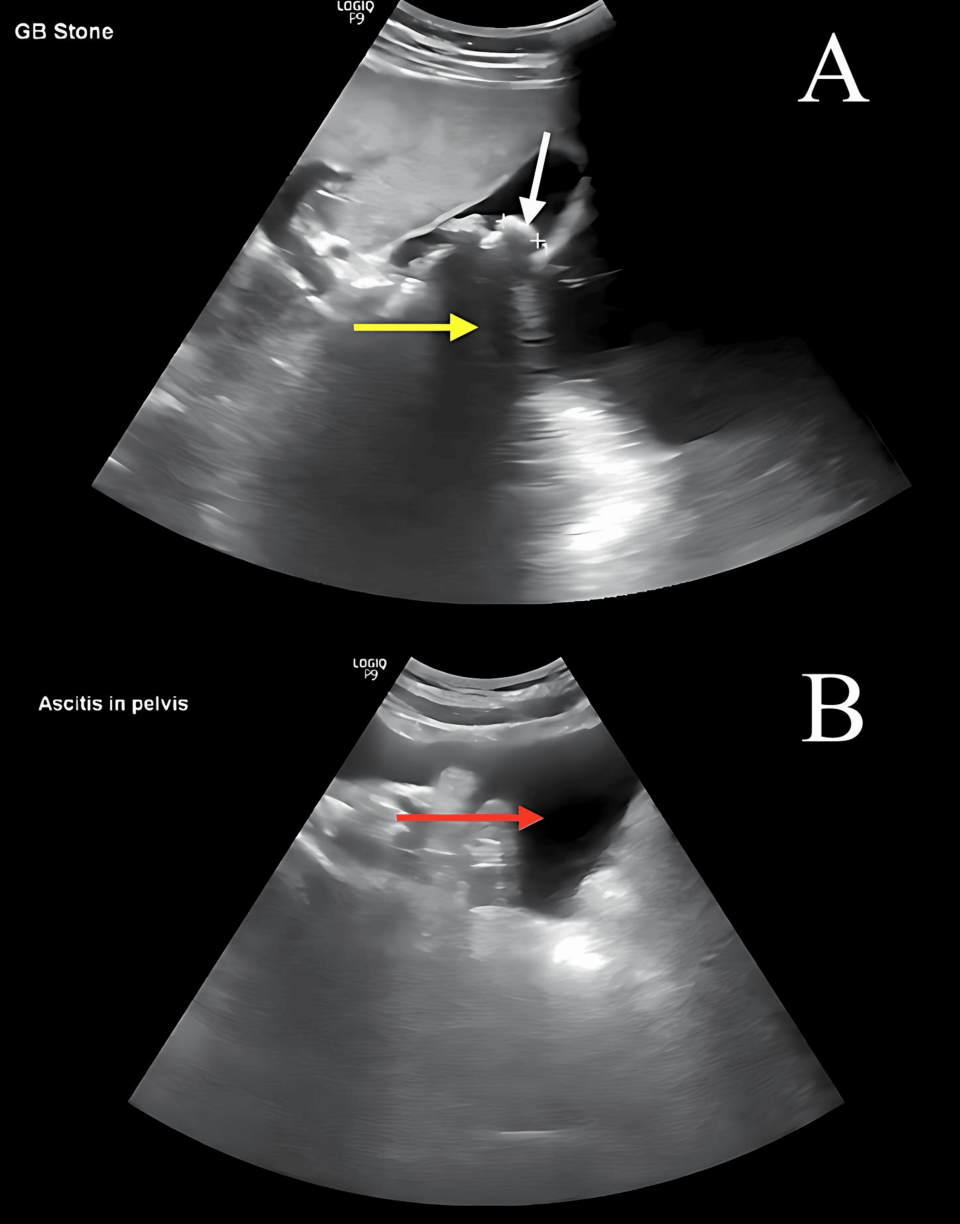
Abdomen and pelvis ultrasound Yellow arrow: posterior acoustic shadow.  White arrow: gallbladder stones. Red arrow: ascites In the pelvis.
